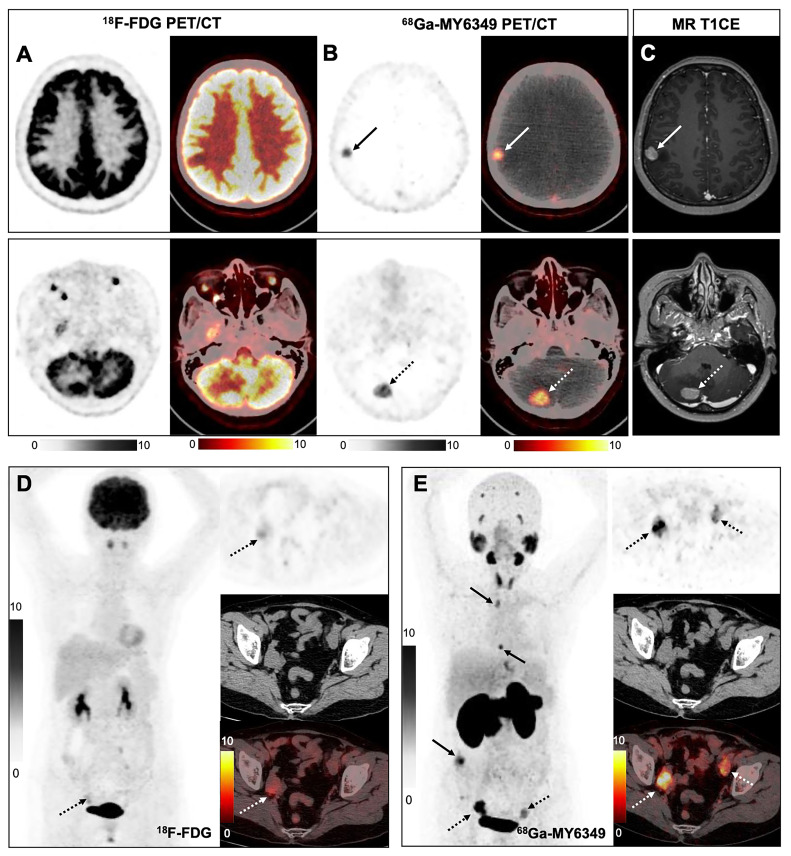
Use of ^68^Ga-MY6349 PET/CT for breast-cancer restaging. (A–C) A 43-year-old woman presented with a persistent headache 5 months after surgery for left breast lobular carcinoma (luminal B, HER2^-^). PET/CT was performed to evaluate possible recurrence or metastasis. Owing to the intrinsically high physiological background in the brain, ^18^F-FDG PET/CT failed to clearly identify any brain lesions (A). In contrast, ^68^Ga-MY6349 PET/CT detected a metastatic lesion in the right frontoparietal lobe (B). Subsequent MRI confirmed the ^68^Ga-MY6349 PET/CT features. (D, E) Another 43-year-old woman presented with progressively rising CEA levels 4 years after surgery for left breast lobular carcinoma (luminal B, HER2^-^). ^18^F-FDG PET/CT showed no definitive hypermetabolic lesions; a mild uptake was observed in the right adnexal region, difficult to distinguish from physiological ovarian activity (D). In contrast, ^68^Ga-MY6349 PET/CT revealed bilateral adnexal metastases (E). Additionally, ^68^Ga-MY6349 PET/CT revealed metastases in the mediastinal lymph nodes, thoracic spine, and mesentery not visualized with ^18^F-FDG PET/CT. Based on these observations, both patients’ treatment plans were revised from routine surveillance to systemic chemotherapy. ^18^F-FDG: ^18^F-fluorodeoxyglucose; CT: computed tomography; HER2: epidermal growth factor receptor 2; MRI: magnetic resonance imaging; PET: positron emission tomography.
